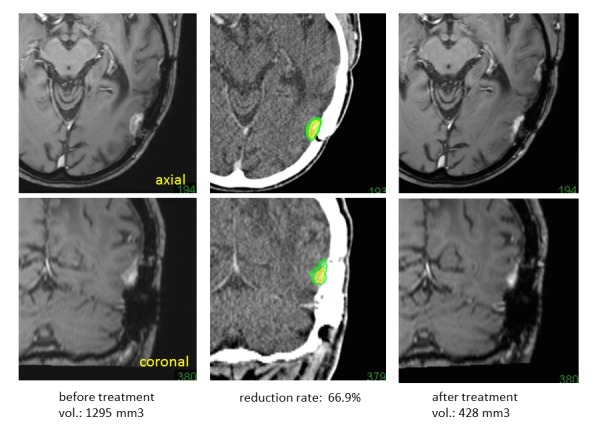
Measurement of tumor reduction rates. MR images fused to thin-sliced CT before radiosurgery (left). Follow-up MR images after treatment (right) also fused to CT (base on images taken during radiosurgey). Both images are able to compare with identical axial (upper line) and coronal (lower line) images using image fusion. The quantitative analysis (of the reduction rate) is also possible from the volume measurements taken before and after treatment (center).
